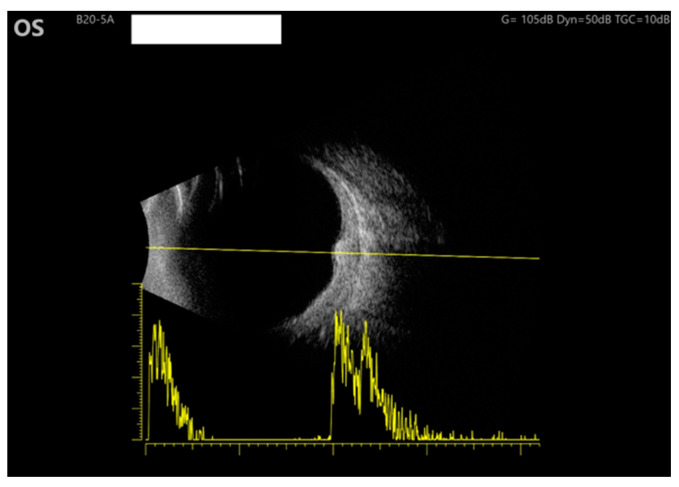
Ultrasound of the LE: B scan demonstrates moderate internal reflectivity through the lesion and diffuse choroidal thickening.
